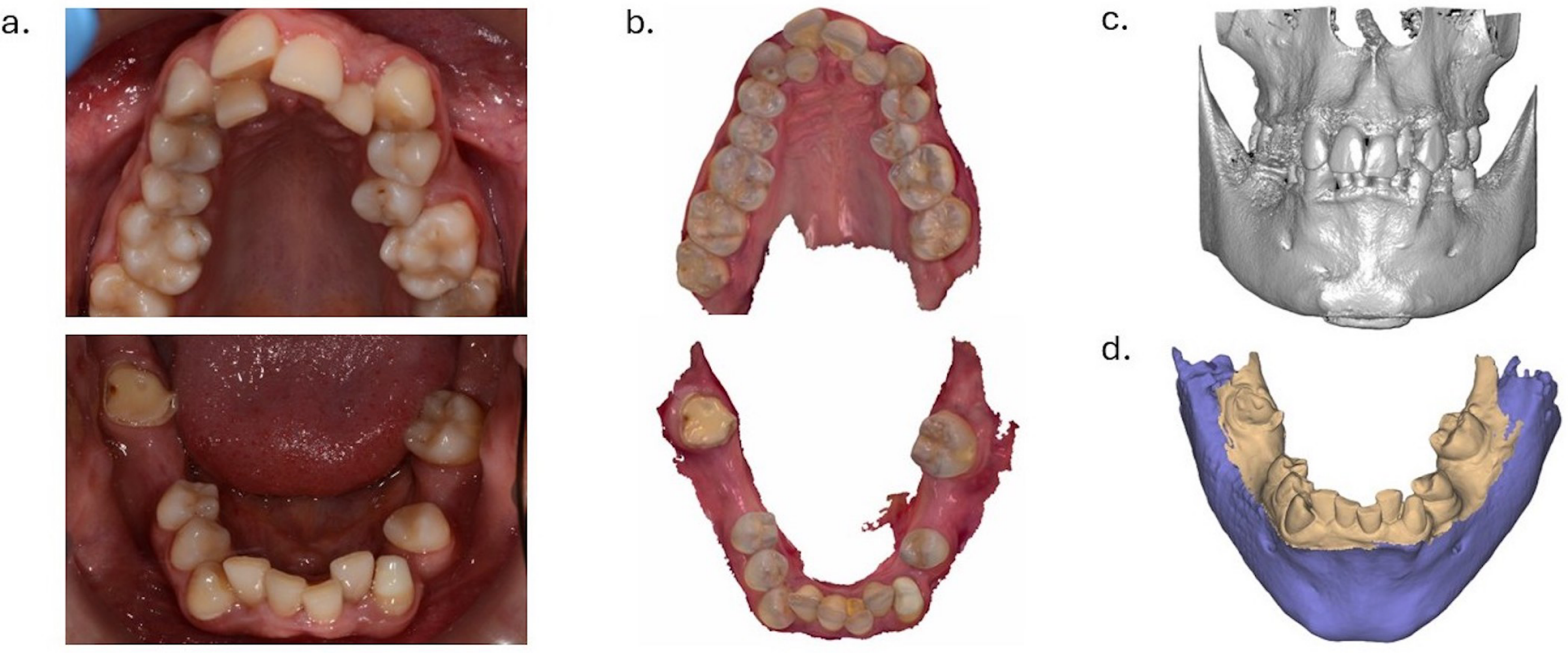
Diagnostic procedures for tooth autotransplantation of a mature tooth in a 27 years old male patient—acquiring clinical, intraoral scan and cone beam computed tomography data to create the virtual patient. (a) Preoperative occlusal aspects of the maxillary and mandibular arch. (b) Intraoral scan. (c) Cone beam computed tomography examination. d. Virtual patient obtained by the superimposition of the intraoral scan file over the CBCT file.
